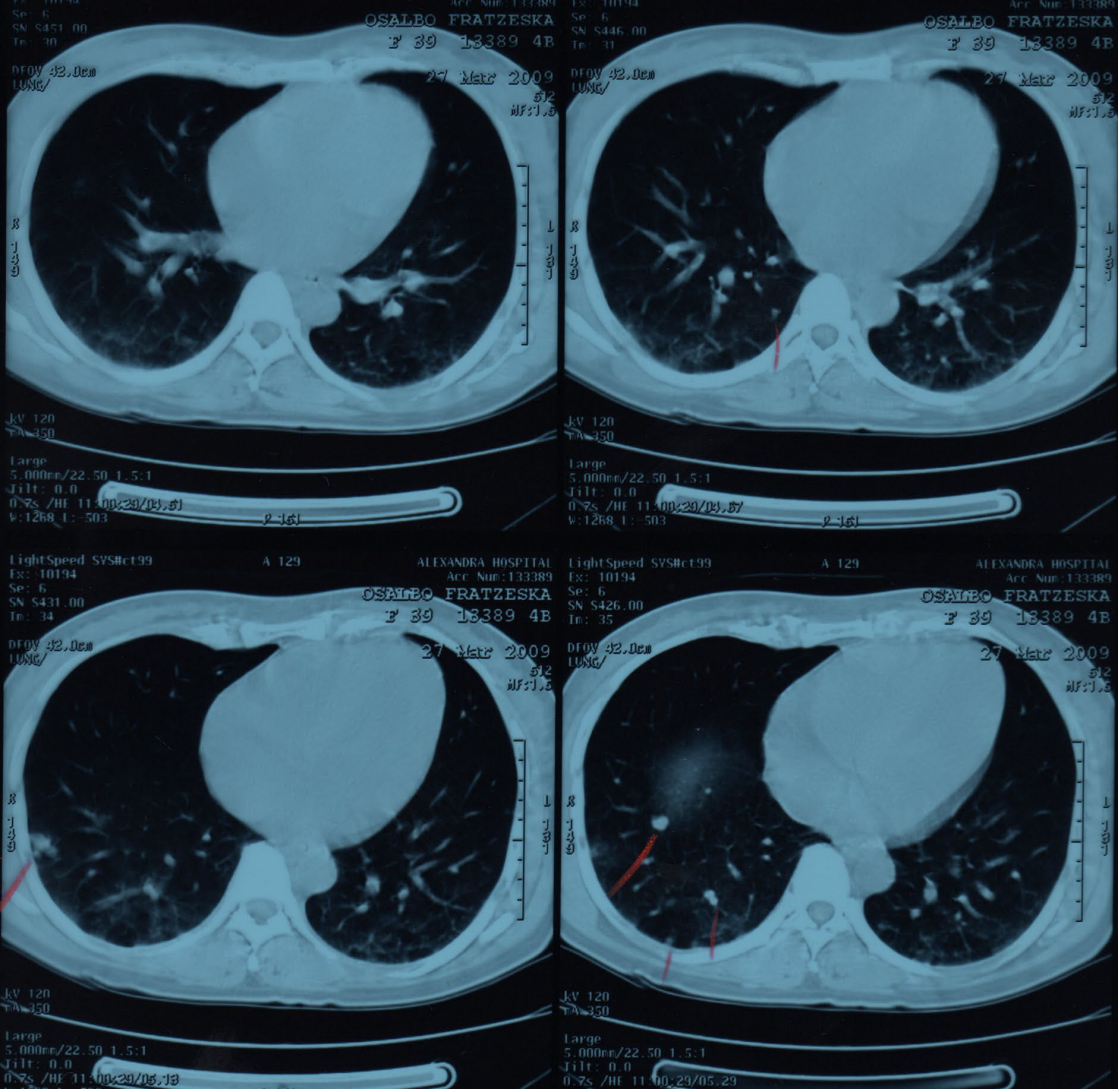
Chest CT showing the multiple metastases of the trophoblastic disease.
